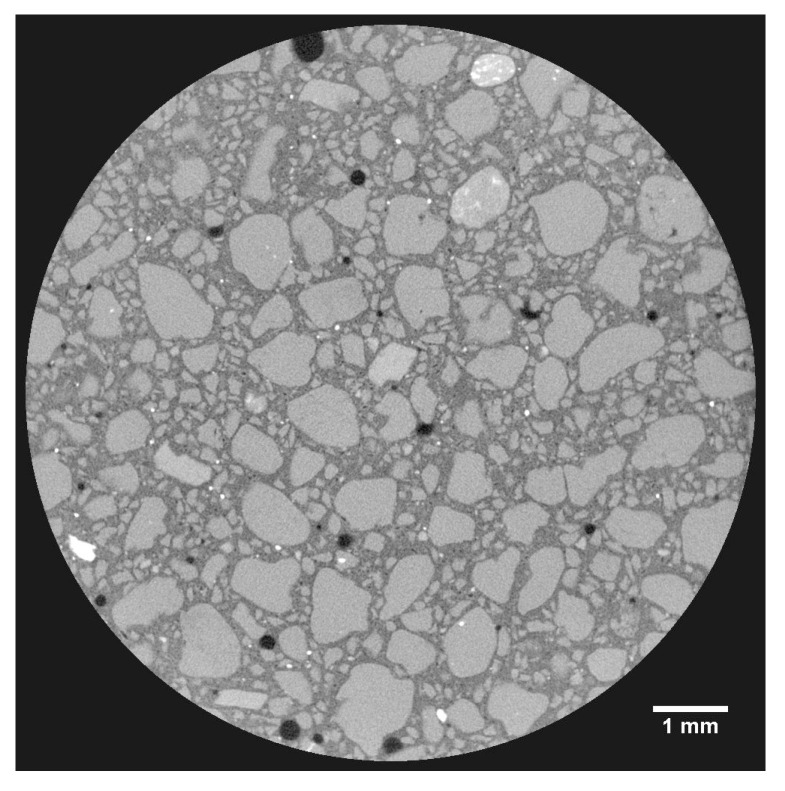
Slice of X-ray tomography for sample NQ.
